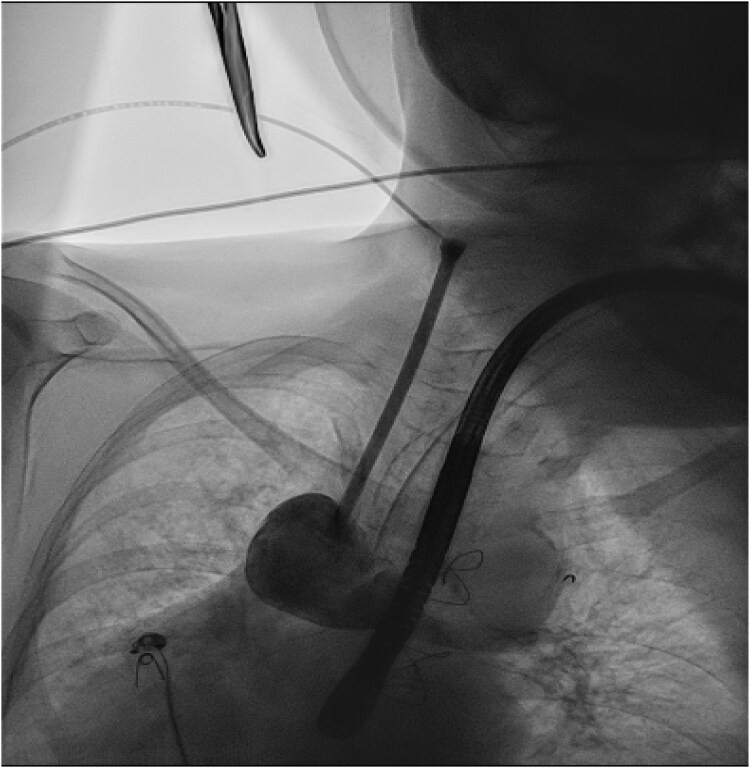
3D TOE echocardiogram image of RF needle puncturing Fontan baffle.
